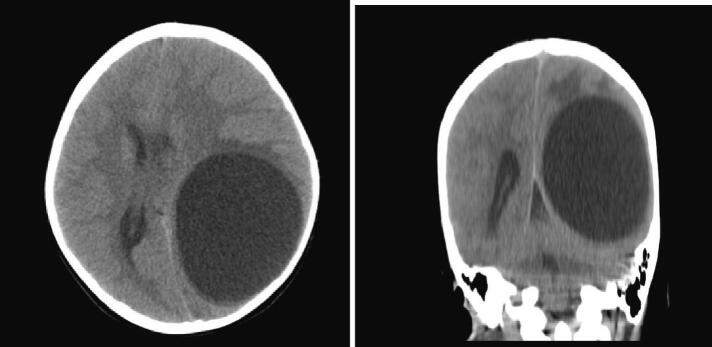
Axial and coronal brain CT Scan showing cystic lesion in left temporo-parieto-occipital region.
